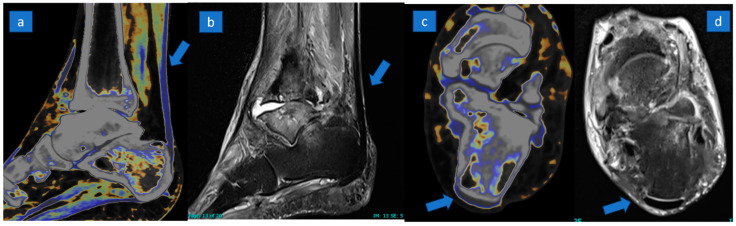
A 57-year-old male with a painful ankle due to rheumatoid arthritis. Normal tendon (arrow) is coded in blue on sagittal (a) and axial (c) super-imposed color-coded maps. The normal appearance of a tendon (arrow) is confirmed on sagittal (b) and axial (d) PD Fat-saturated MR images.
